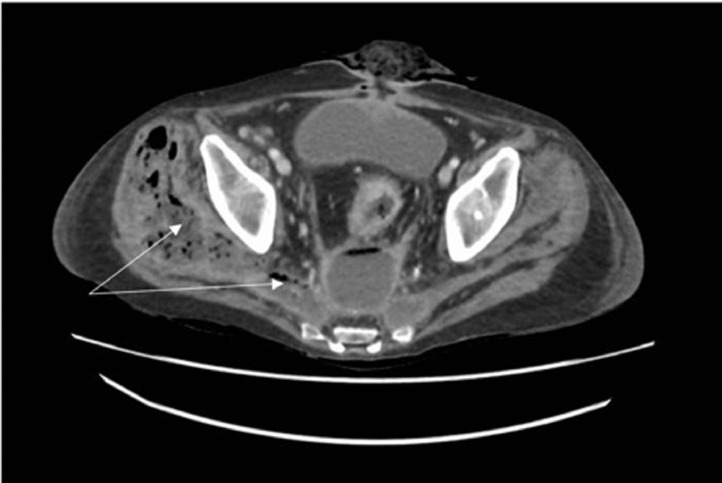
Computed tomography of the patient at presentation to hospital. Arrows indicate to gas observed in the gluteal soft tissues, which tracks into the pelvis. Communicating gluteal collection and sacral collection are also observed and highlighted.
